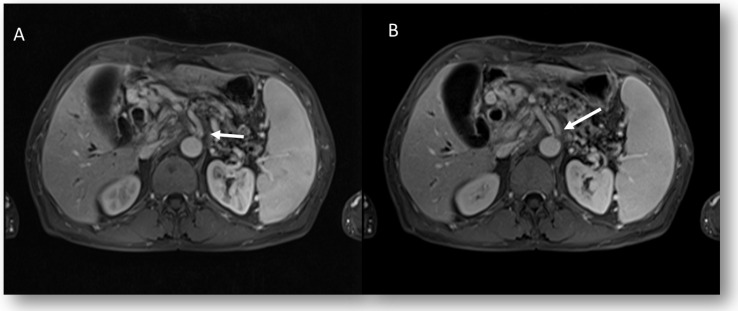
Pancreatic cancer patient. MRI staging assessment (arterial (A) and portal (B) phase of contrast study). The arrows show right hepatic artery origin from the superior mesenteric artery.
